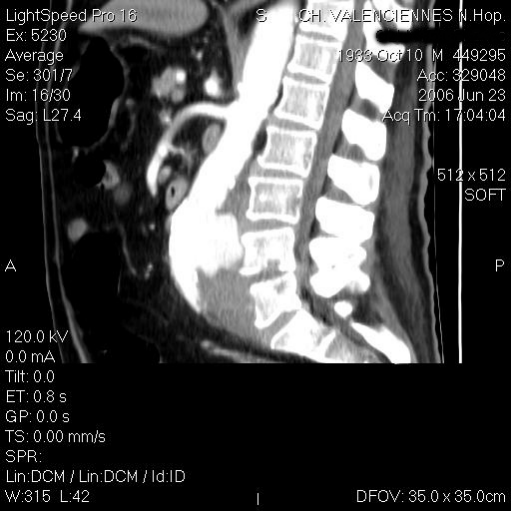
A sagittal CT image of the lumbar area with contrast reconstruction showing abdominal aortic aneurysm.
